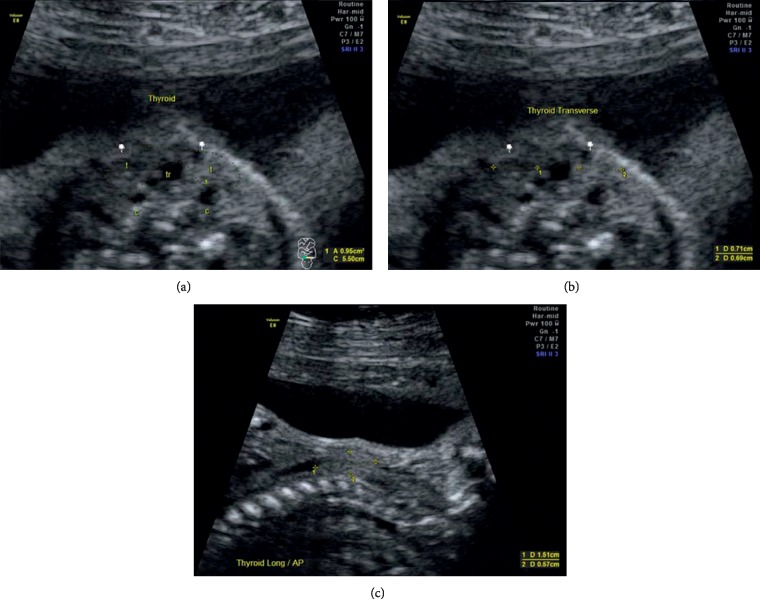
Ultrasound scan showing measurements of fetal thyroid lobe diameters in a 31-week gestation. (a) Transverse cut of the neck showing the thyroid lobes (t) around the trachea (tr) and in front of the carotid arteries (c). (b) Transverse diameters of both lobes of the fetal thyroid. (c) Longitudinal and anteroposterior diameters of the fetal thyroid lobe in the sagittal plane.
